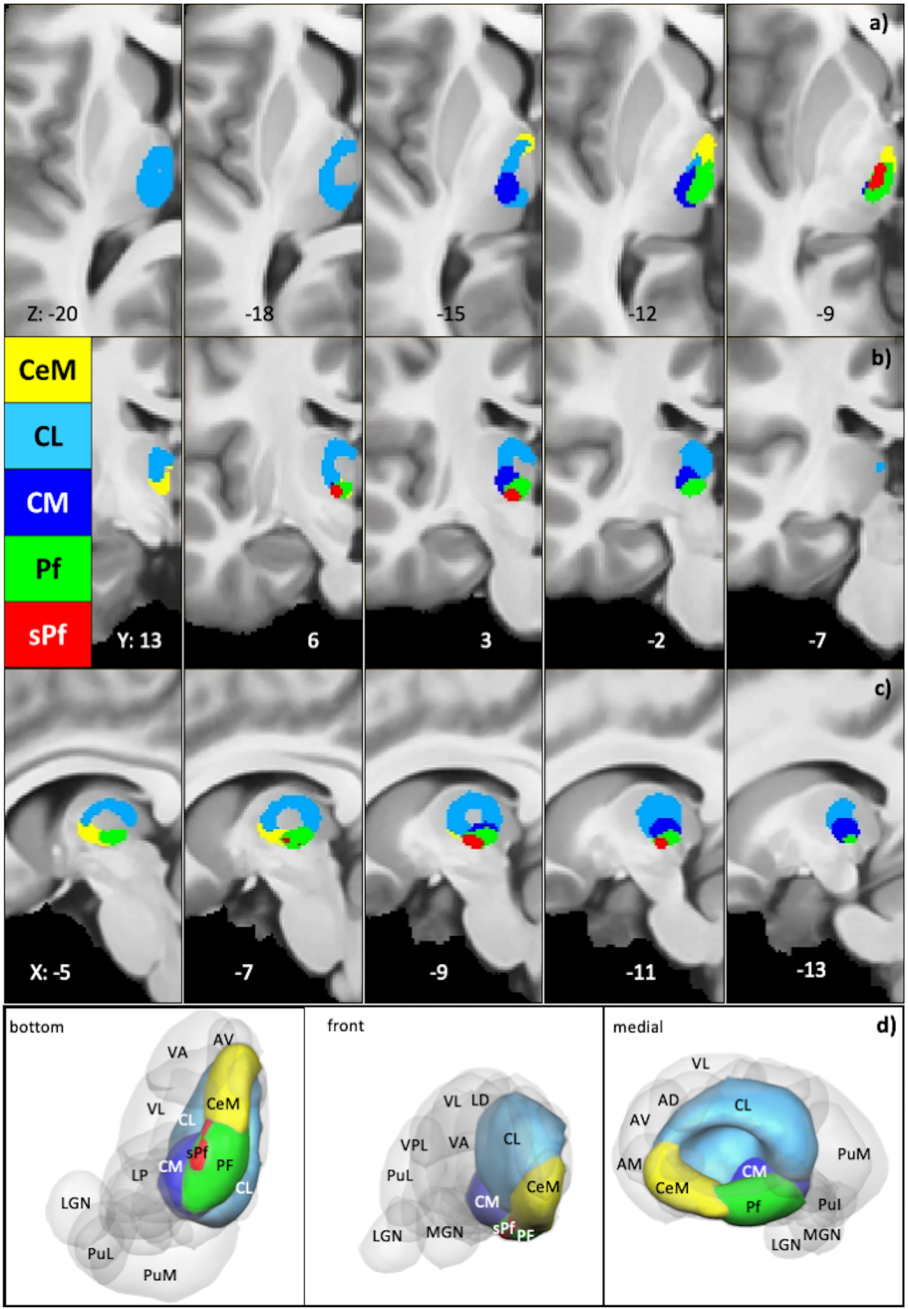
Intralaminar Nuclei Location Anatomy: location depiction of intralaminar nuclei within MRI 2D and 3D visualization of Intralaminar nuclei. (a–c) 2D maps show each intralaminar nuclei with its color code (depicted in the figure) on axial, coronal, and sagittal views. (d) 3D maps show each nucleus within the thalamus, with the whole brain perspectives from the bottom, front, and medial sides.
